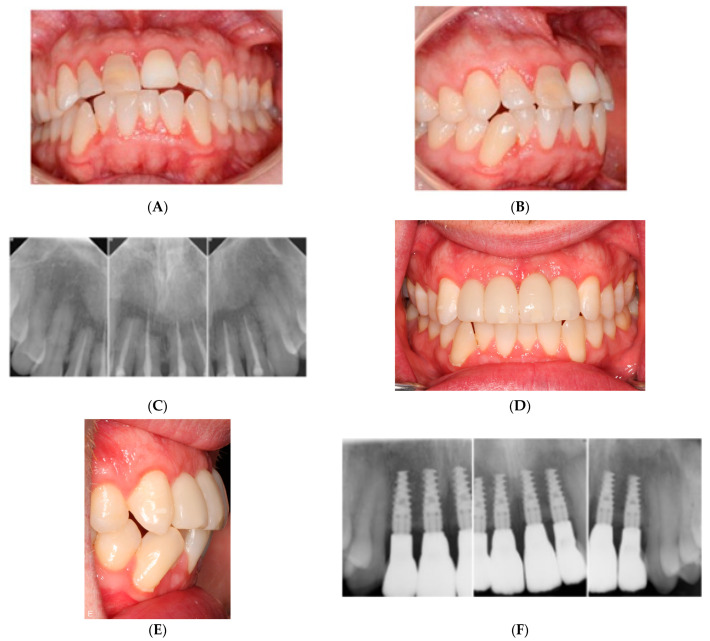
Trauma case. Teeth 12–22 avulsed due to bicycle trauma. Regio 12–22 reconstructed with zirconia crowns placed on four titanium implants (Nobel Active NP, 11.5 mm) using a virtual planning technique. (A) Anterior maxilla frontal view, preoperative status. (B) Anterior maxilla lateral view, preoperative status. (C) Intraoral radiographs region 12–22, preoperative status. (D) Anterior maxilla frontal view, postoperative status. (E) Anterior maxilla lateral view, postoperative status. (F) Intraoral radiographs region 12–22, postoperative status.
